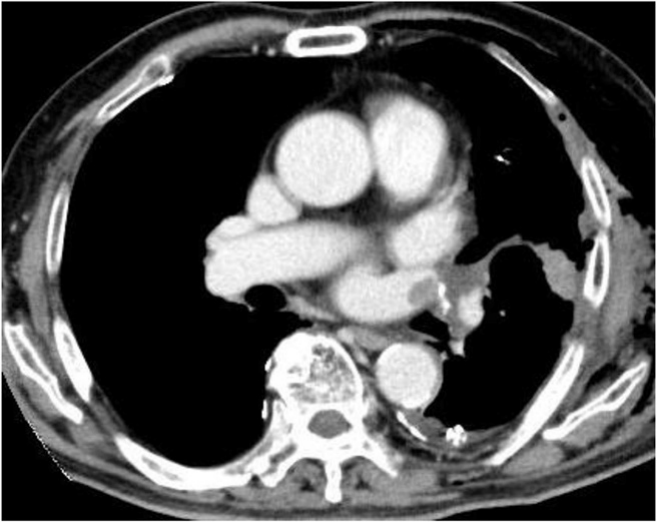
Thrombosis in the left superior pulmonary vein stump. In the patient who showed SEC in the left superior pulmonary vein stump on intraoperative ultrasonography, thrombosis in the stump is seen on contrast-enhanced computed tomography.
